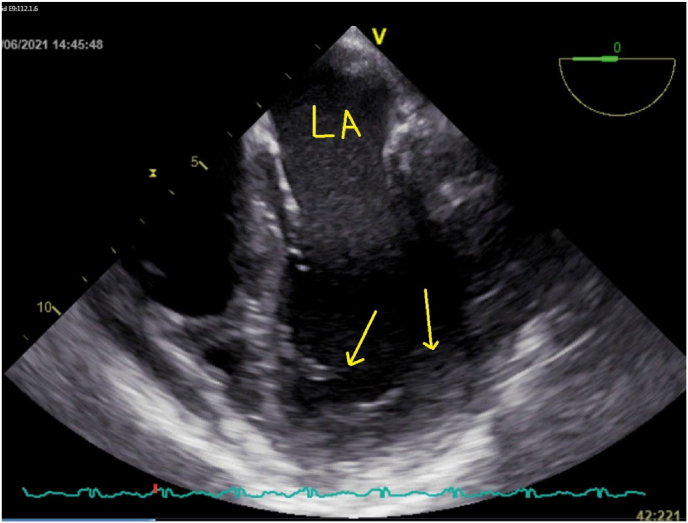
2-dimensional echocardiogram showing filamentous fibrinous structural along pericardial floating into the fluid.
